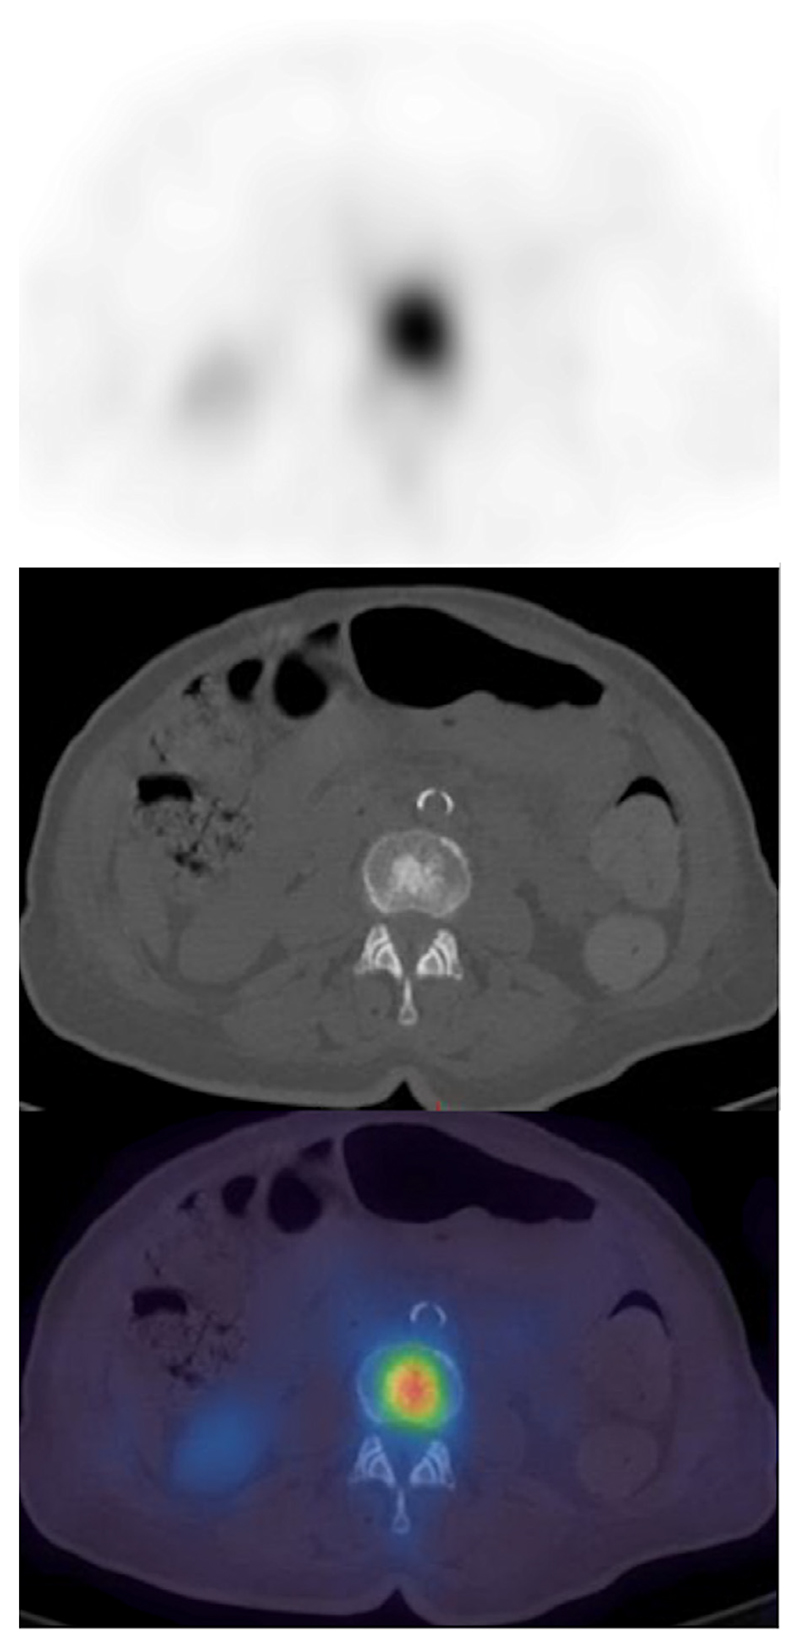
Planar ^99m^Tc-MDP bone scan (a) showing a left humeral metastasis and an indeterminate focus of activity in the mid lumbar spine (arrows). SPECT/CT images (b) localize the abnormal uptake to the vertebral body and show corresponding sclerotic appearances in keeping with a metastasis.
